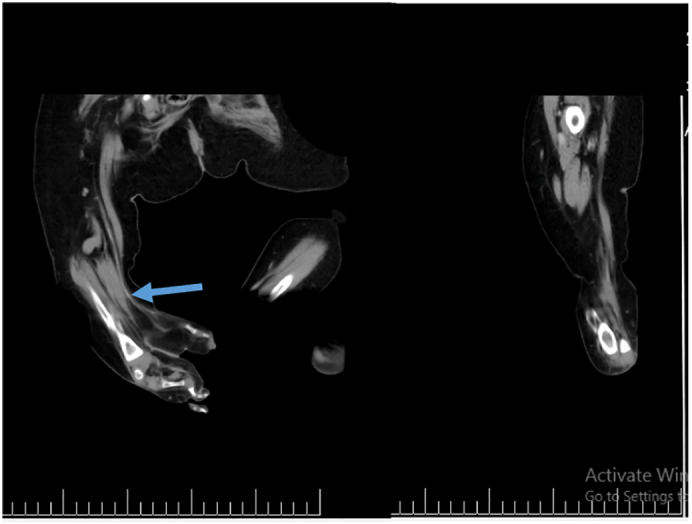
CT angiography. Blue Arrow: bifurcation of normal and duplicated artery to the duplicated foot. (For interpretation of the references to colour in this figure legend, the reader is referred to the web version of this article.)
